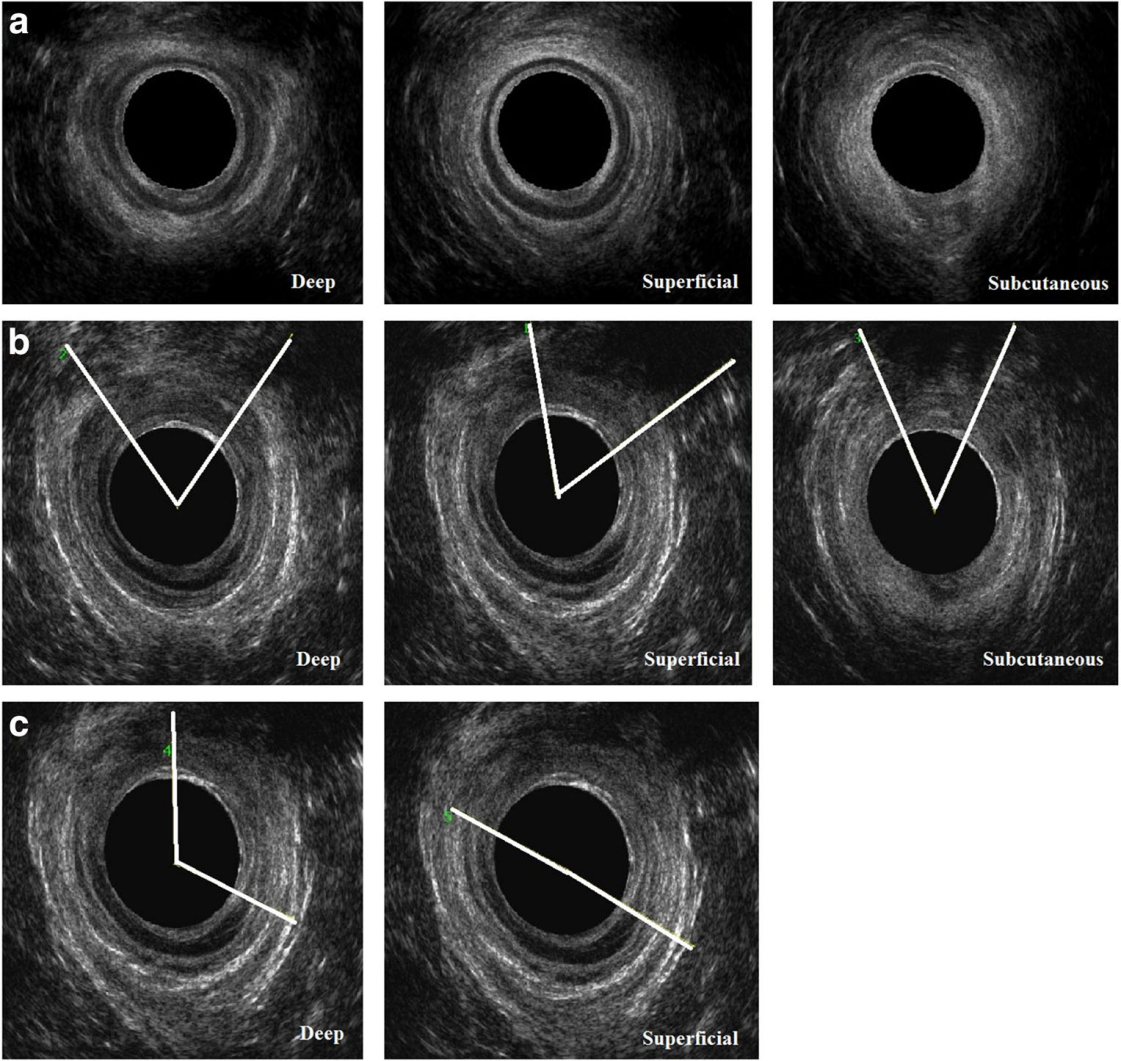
Endoanal ultrasound of the anal sphincters: slices at the deep (*left column*), superficial (*middle column*) and subcutaneous levels (*right column*). a Intact sphincters. b Defect in the external anal sphincter (hypoechoic ring) indicated by the angles. c Defect in the internal anal sphincter (hyperechoic ring) indicated by the angles
